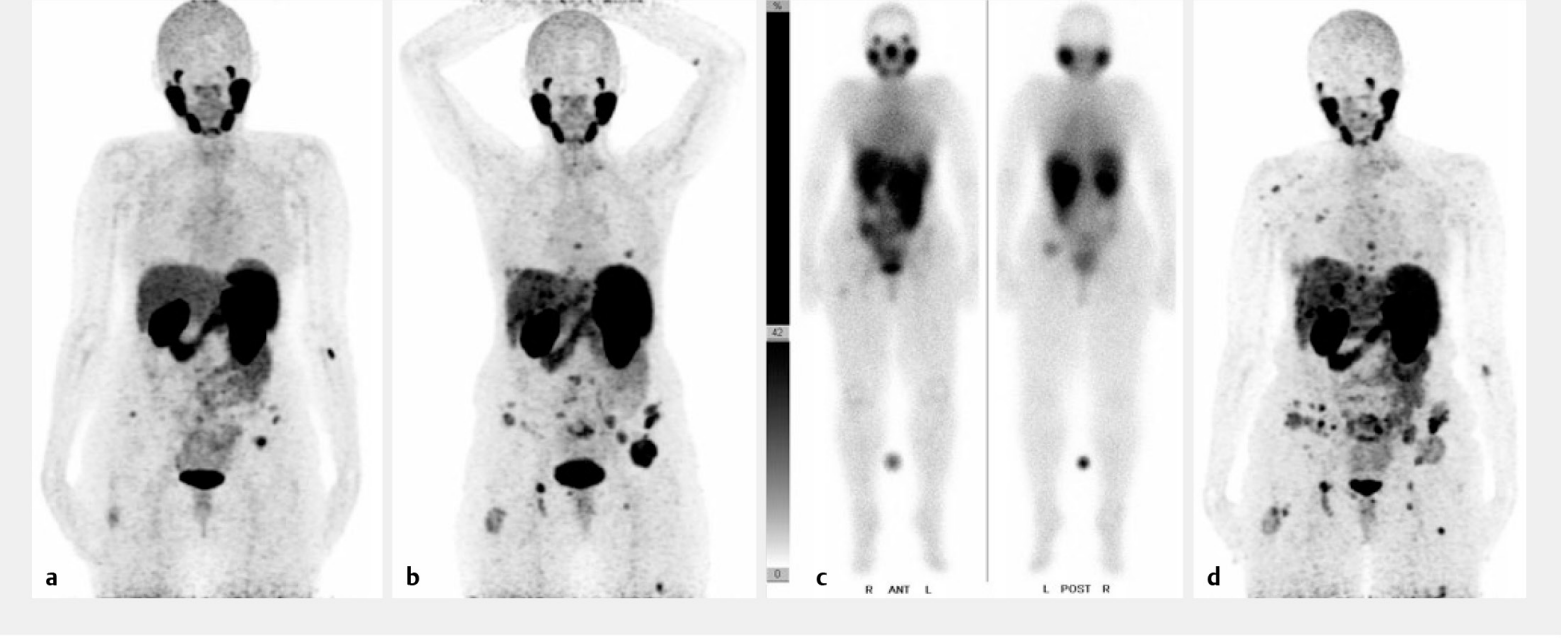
A 50-year-old female patient with metastasized leiomyosarcoma has received one application of 6.0 GBq [^177^Lu]Lu-PSMA-617. This figure was obtained from Jüptner et al.^
